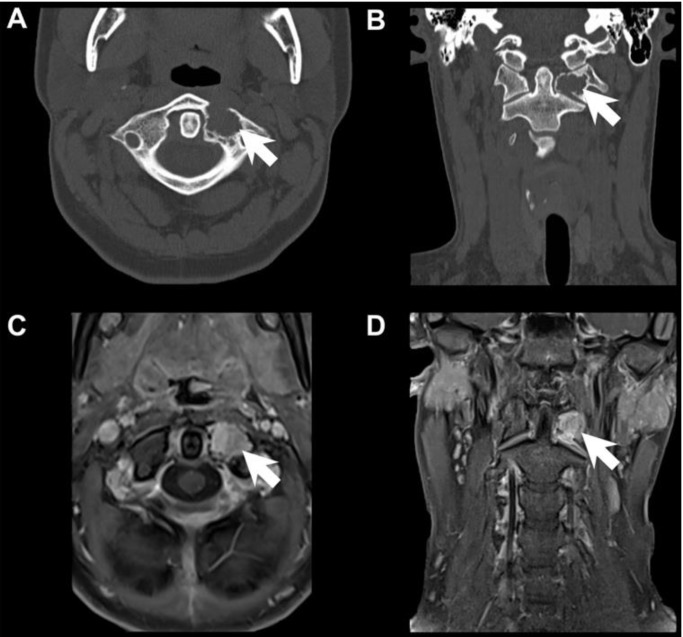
Pre-Operative Imaging Studies. CT of cervical spine, axial view, demonstrating the radiolucent, ovoid-shaped, lytic lesion (white arrow) arising in the left lateral mass of C1 between the anterior tubercle and the transverse process (A). CT of cervical spine, coronal view, demonstrating the lesion (white arrow) (B). Post-contrast MRI T1, axial view, demonstrating an enhancing osseous lesion (white arrow) at the left lateral mass of C1 with cortical breach and extension into the left lateral atlantodental joint space (C). Post-contrast MRI T1, coronal view, of the lesion (white arrow) (D).
